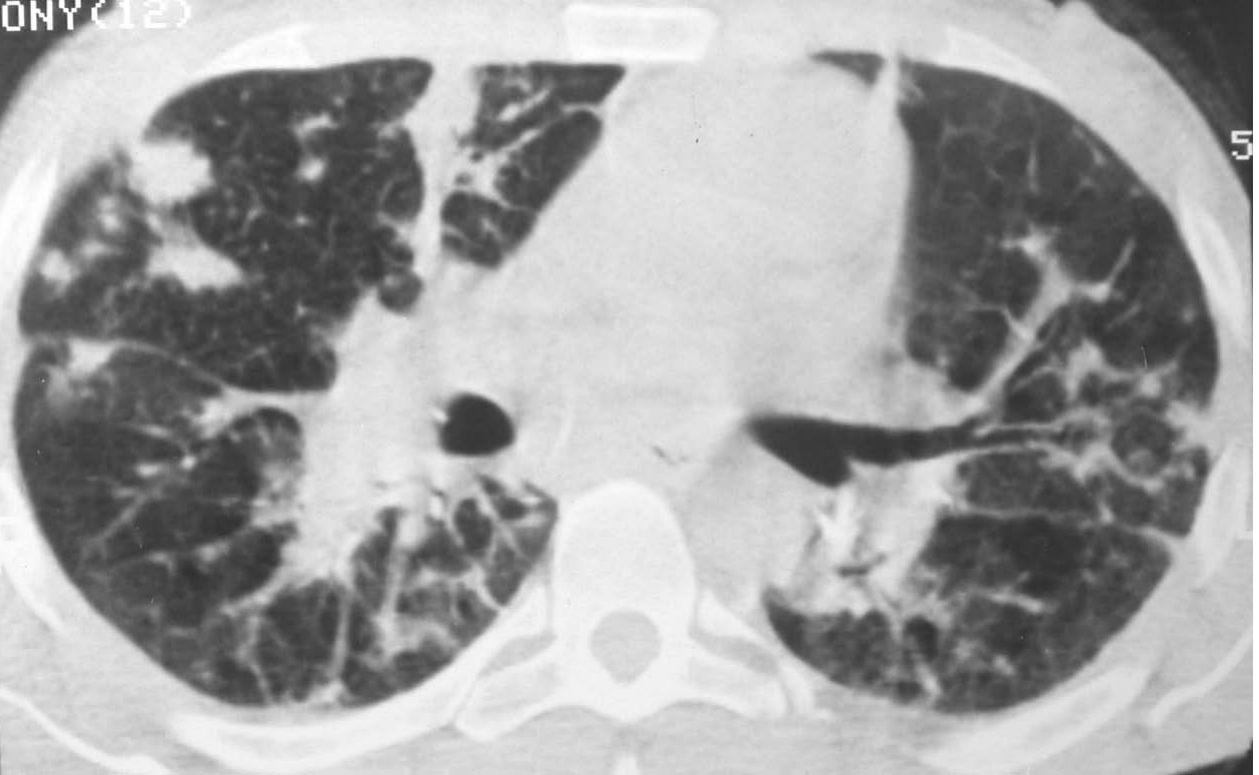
High-resolution CT scan at the level of the main bronchi of a patient with pulmonary KS, showing diffuse ill-defined large nodules and paracardiac peribronchovascular thickening.
